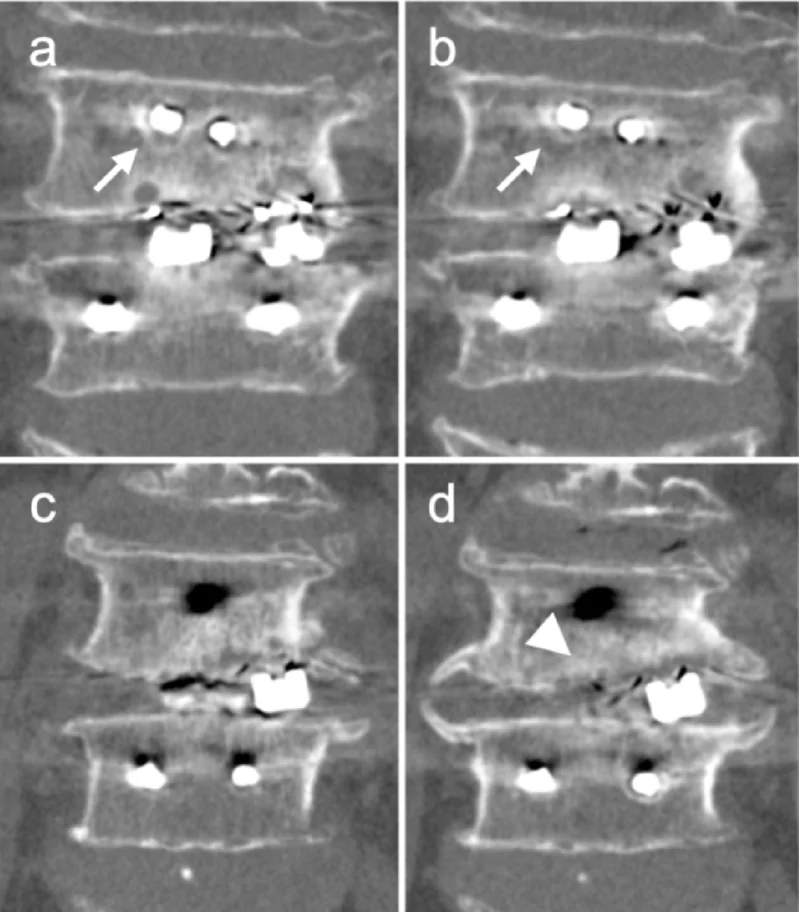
Temporal changes in the radiographic appearance. (a, c) CT images obtained on postoperative day 177 demonstrating a radiolucent zone around the pedicle screw (arrow). No evidence of bone bridging is observed at this time point. (b, d) CT images obtained on postoperative day 555 showing a reduction in the size of radiolucent zone (arrow) to below the 1-mm threshold used to define loosening. Evidence of bone bridging (arrowhead) is also observed.
